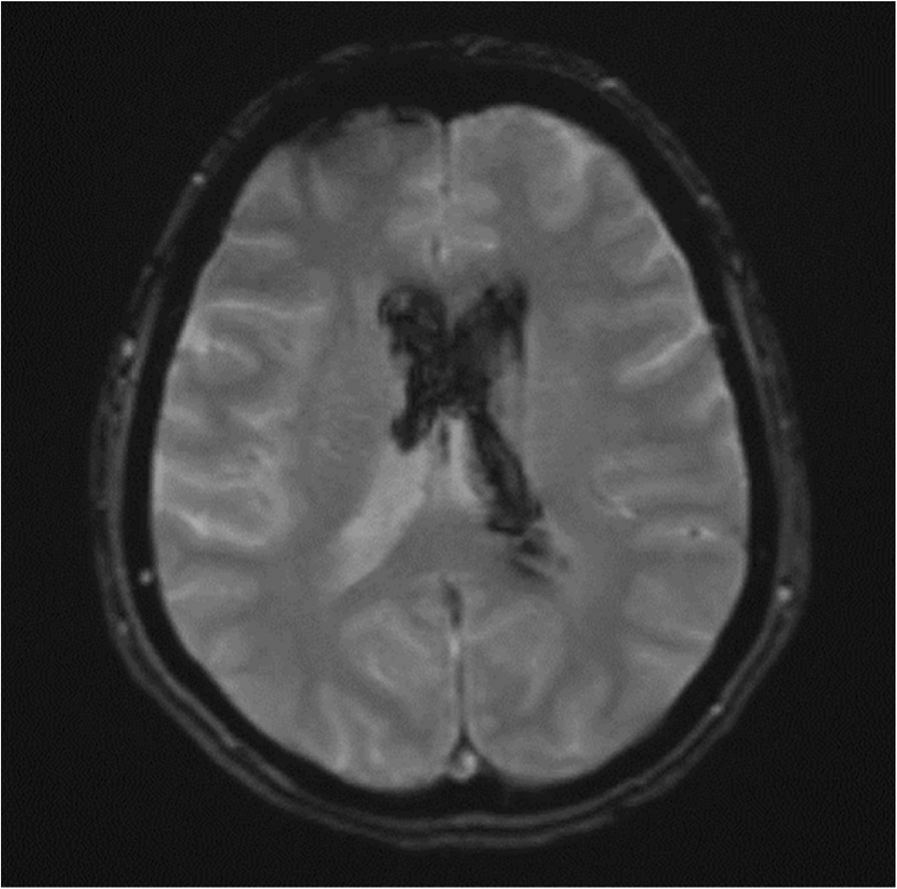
MRI brain [fast low angle shot (FLASH) sequence] demonstrated intraventricular hemorrhage; no causative underlying vascular lesion was identified
